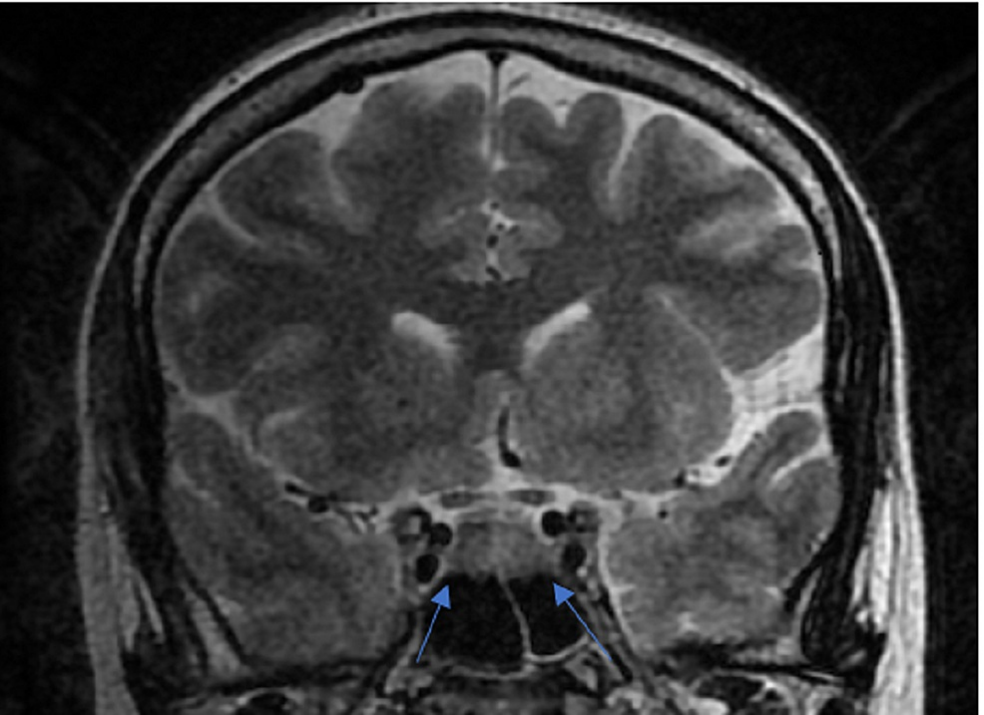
Coronal T2-weighted image showing two isointense lesions (arrow), with hyperintense normal pituitary tissue separating both the lesions.
